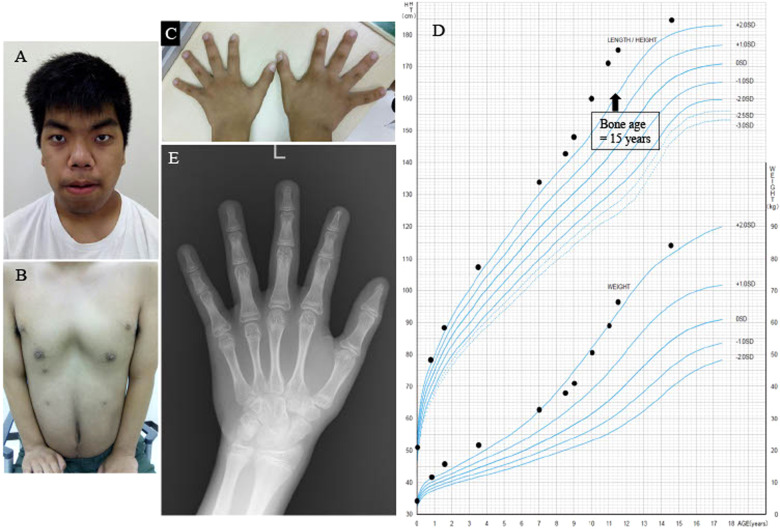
Images of the face, supernumerary nipples, hands, growth curve, and radiography of the left hand. a The patient exhibits macrocephaly. b The patient shows bilateral supernumerary nipples. c The patient’s hands show hypoplastic nails. d Growth curve showing accelerated growth velocity around the age of 10 because of early onset of puberty. e A radiograph of the left hand shows a bone age of 15 years at the chronological age of 11.5 years. Written informed consent was obtained from the patient’s family for publication of this clinical report and accompanying images.
